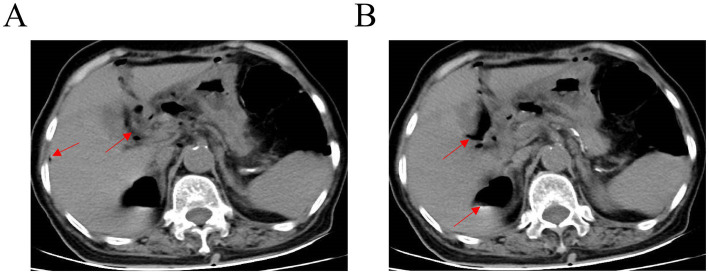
Abdominal CT images of the patient (A,B). Abdominal CT scan showing free gas (red arrow) in the abdominal cavity.
